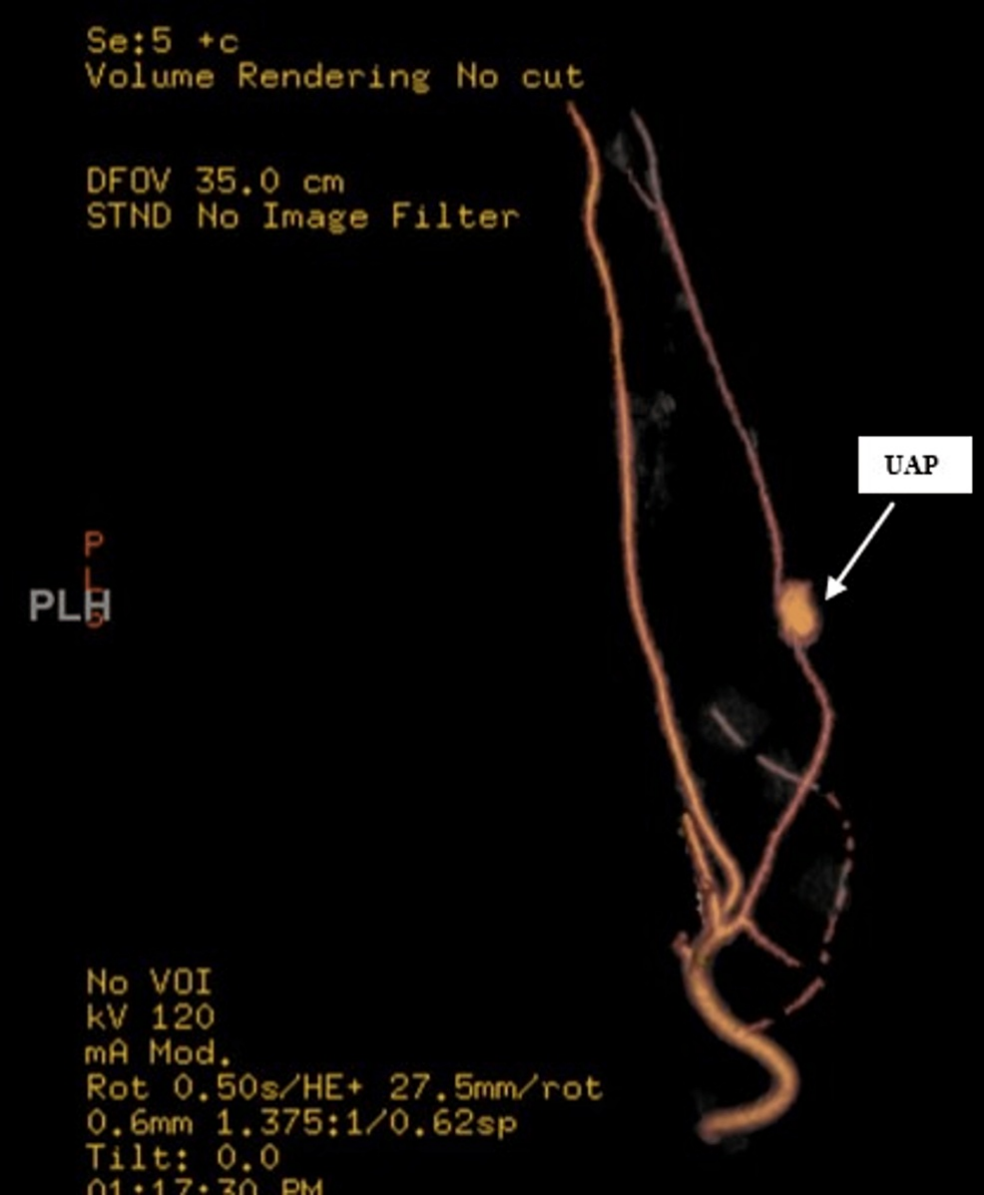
CTA demonstrating focal collection concerning for pseudoaneurysm CTA: computed tomography angiography
